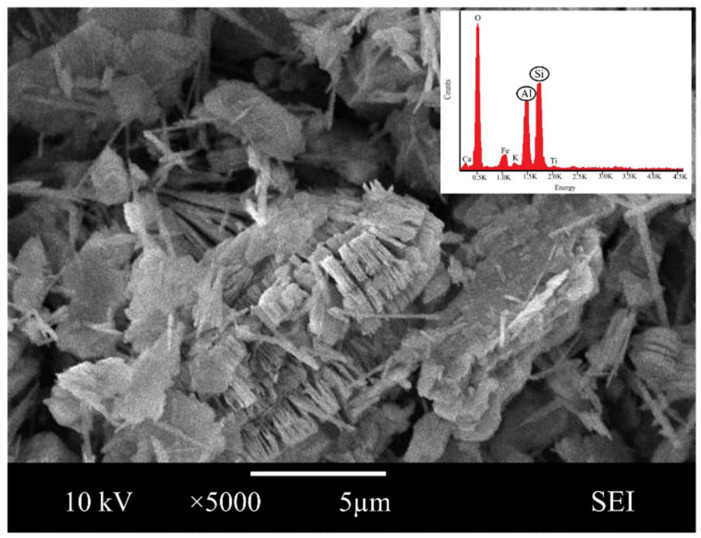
SEM images and energy dispersive X-ray analysis (EDX) of metakaolin.
